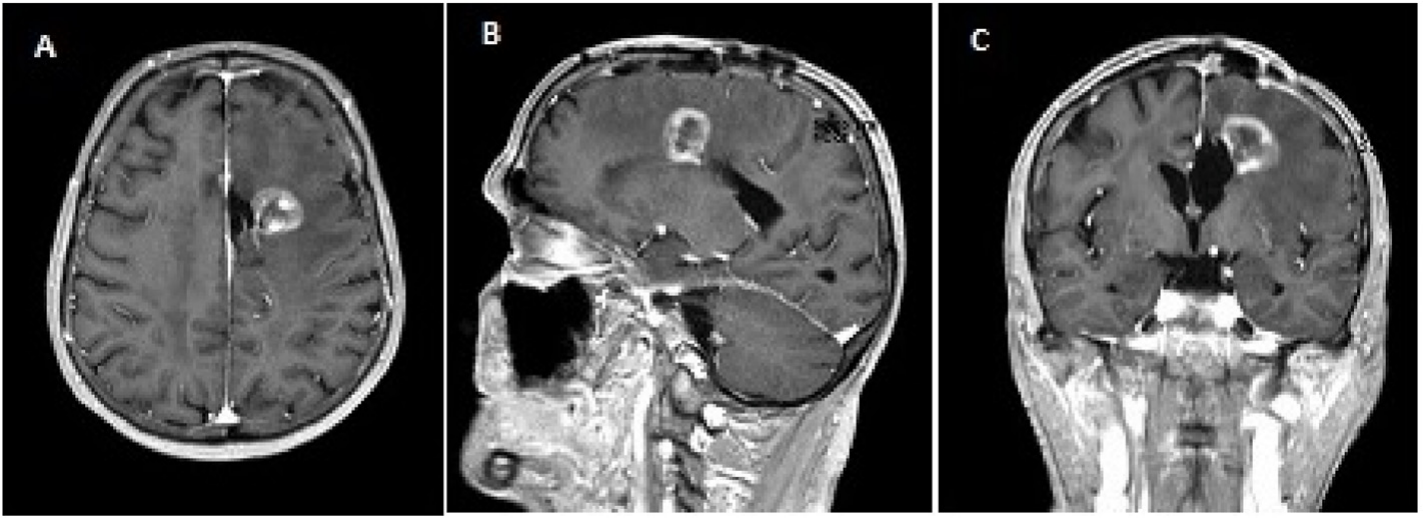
rGBM case - A 55-years old male presents with recurrent glioblastoma, six months after surgical resection and temozolomide/radiotherapy (Stupp protocol). No targetable mutations were found. Patient has a Karnofsky Performance Score of 90. Current MRI is shown. Possible treatment choices were: repeated resection, second line chemotherapy, best supportive care.
